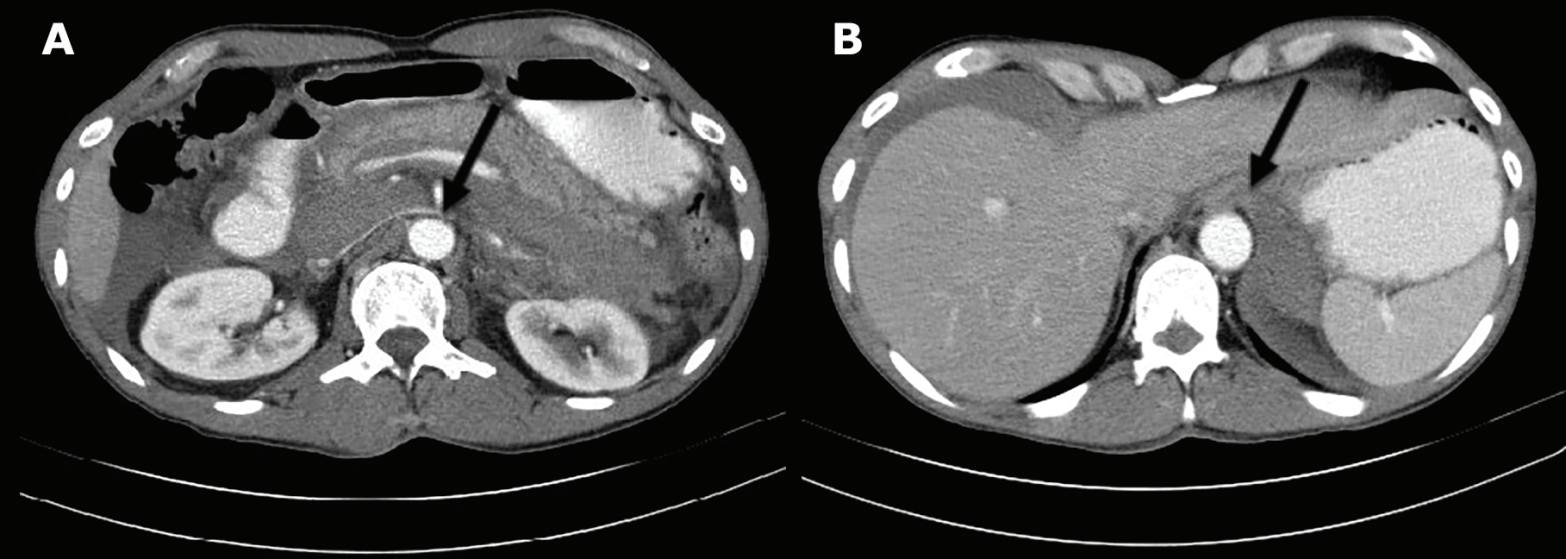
Axial computed tomography (CT) of the abdomen demonstrating retroperitoneal bleeding in the patient (A; Arrow); axial CT-scan of the abdomen demonstrating free air around the liver (B; Arrow)
